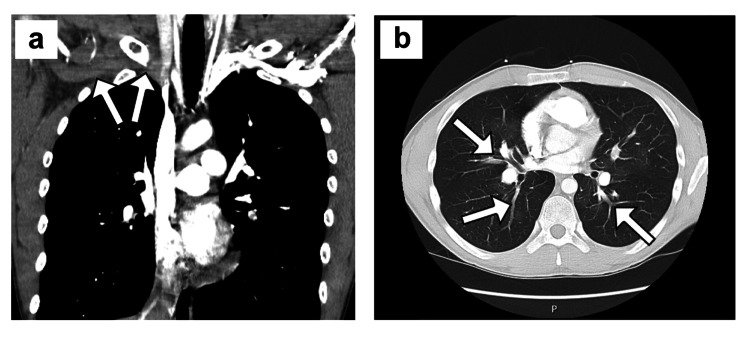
Initial imaging in case 1 a) Coronal view of chest CTA showing right axillary-SCV thrombosis (white arrows). b) Cross-sectional view of chest CTA showing segmental pulmonary artery branch thromboembolism (white arrows). CTA: computed tomography angiography; SCV: subclavian vein
